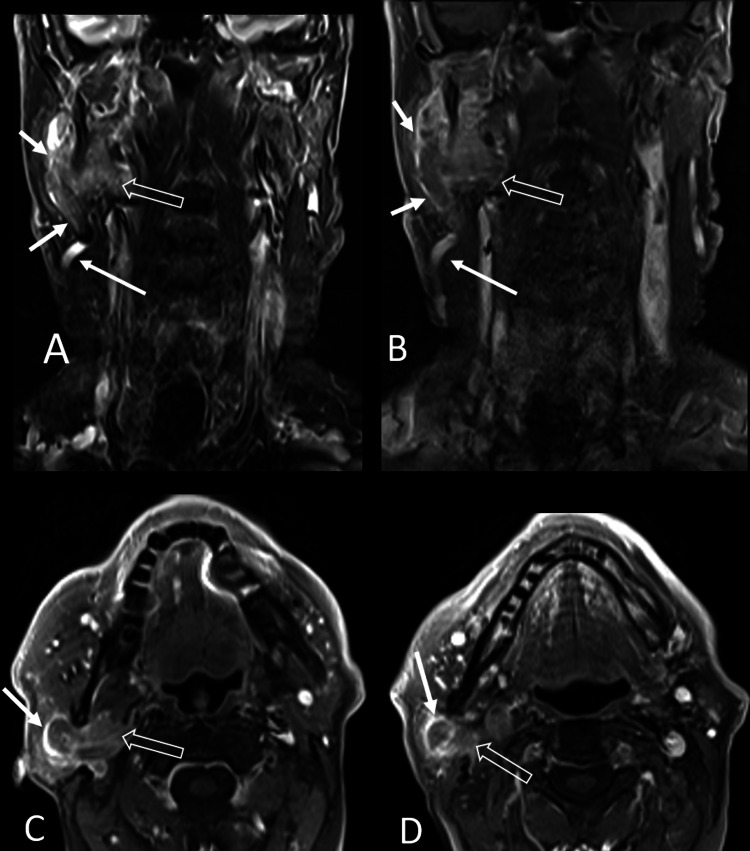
MRI of the neck showing right parotid gland myoepithelial carcinoma. (A) Coronal T2 WI fat-suppressed. (B) Coronal T1 fat saturation, contrast enhancement. (C-D) Axial T1 WI fat saturation, contrast enhancement, higher and lower plane. A lobulated, well-defined mass is noted affecting the superficial and deep lobes of the right parotid gland with high signal on T2WI and strong post gadolinium enhancement (open arrows). Adjacent and lateral to the mass, there is a vertically oriented tubular structure corresponding to a dilated retromandibular vein expanded by intraluminal tumor thrombus (short arrows). Caudally, this vein is in continuity with the external jugular vein (long arrows). WI: Weighted imaging.
